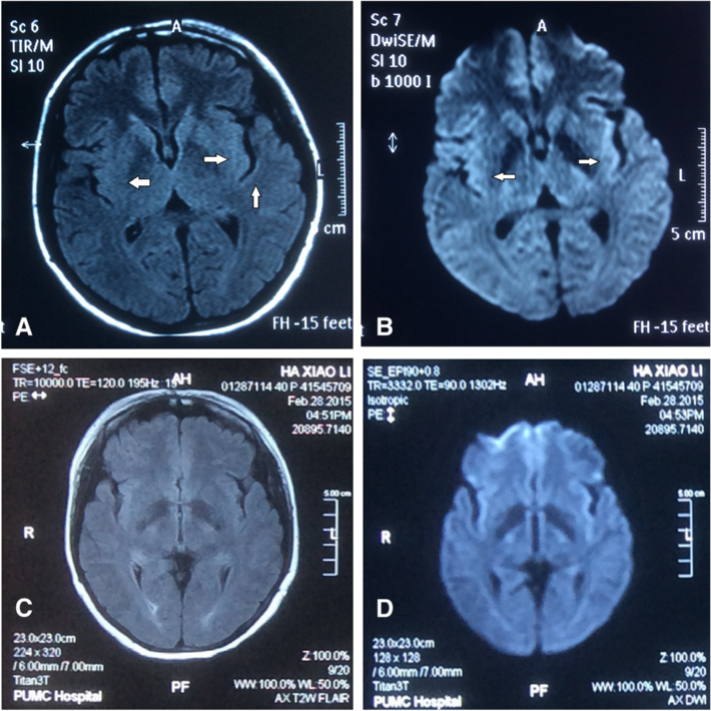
Cranial MRI of our patient. Diffusion-weighted magnetic resonance imaging (DWI) (a) and the corresponding plane in fluid-attenuated inversion recovery (FLAIR) (b) showed bilateral hyper-intensity of the medial temporal lobe, insular lobe and basal ganglia (arrows). Repeated MRI were normal in February 28, 2015. (c, d)
